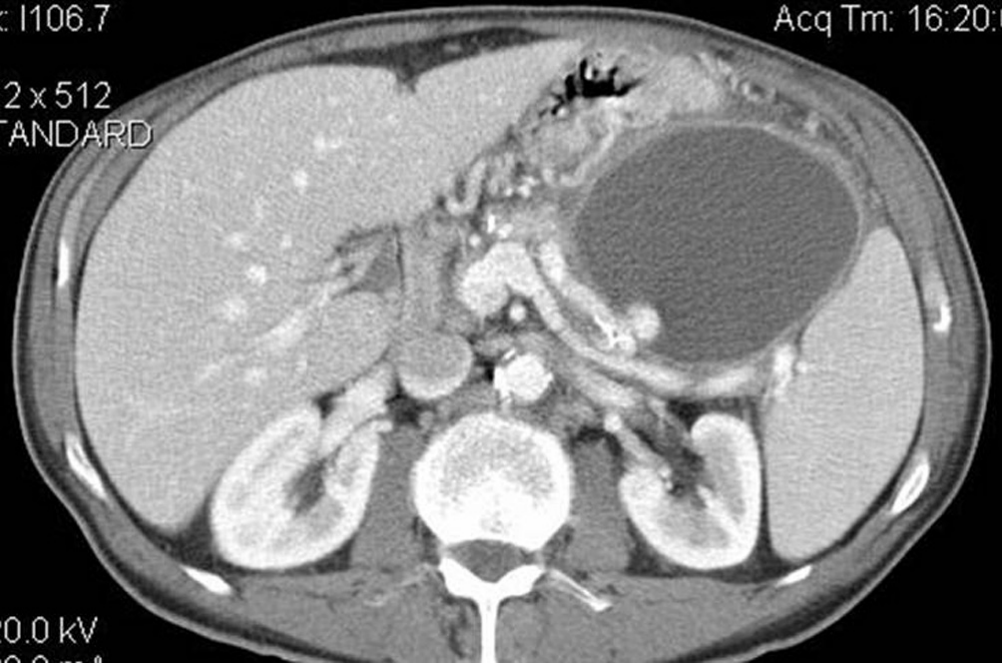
Arterial phase CT shows arterial enhancement pattern of the vascular lesion. Supected diagnosis: splenic artery pseudoaneurysm.
